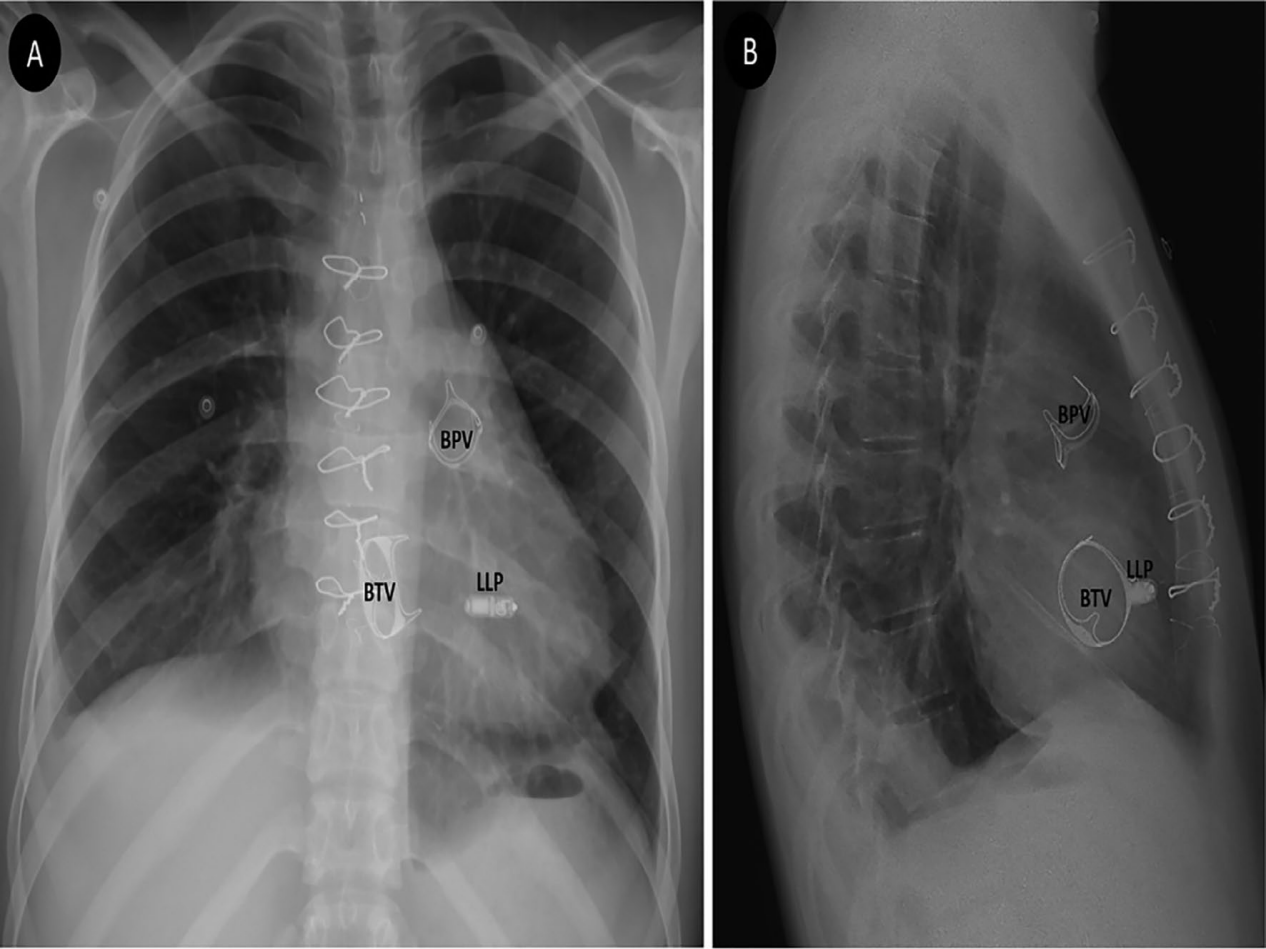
(A) Posteroanterior and (B) lateral chest radiographic views the day after implant. Bioprosthetic tricuspid valve (BTV), bioprosthetic pulmonary valve (BPV), and leadless pacemaker (LLP) are clearly shown in each view.
